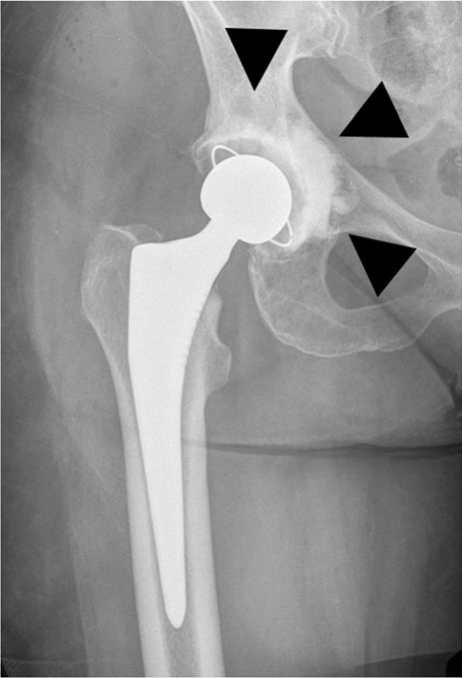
AP radiograph. Cemented total hip arthroplasty. In a reverse hybrid cemented arthroplasty, the acetabular cup is fixed with cement (*arrowheads*)
